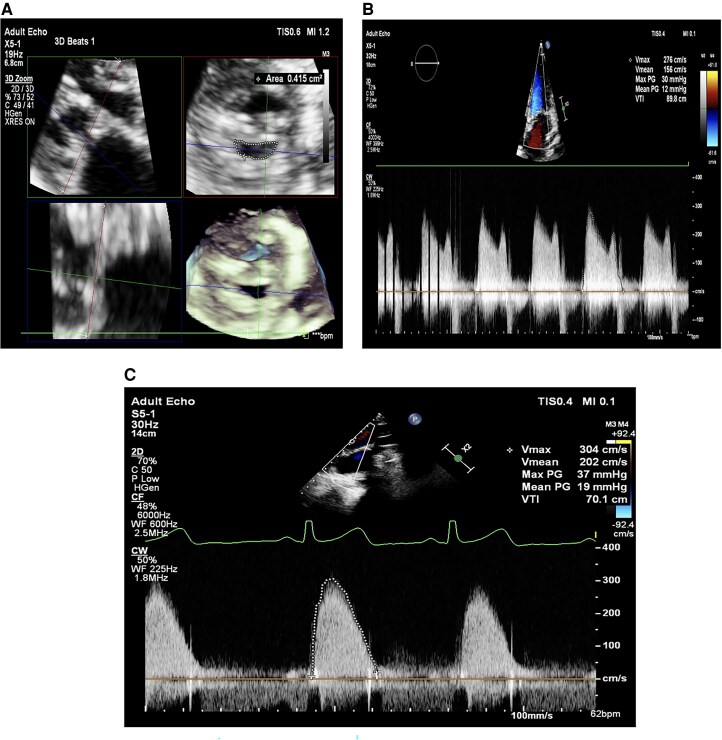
(*A*) Baseline multiplanar three-dimensional reconstruction of the mitral valve orifice on transthoracic echocardiography demonstrating an effective valve area of 0.41 cm^2^, suggesting critical mitral stenosis (upper right segment). (*B*) Continuous wave Doppler study across the mitral valve demonstrating a severe mean transvalvular gradient of 12 mmHg. (*C*) Continuous wave Doppler across the aortic valve in the right parasternal view demonstrating a mean transvalvular gradient of 19 mmHg and a peak velocity of 3.04 m/s suggestive of mild aortic stenosis.
